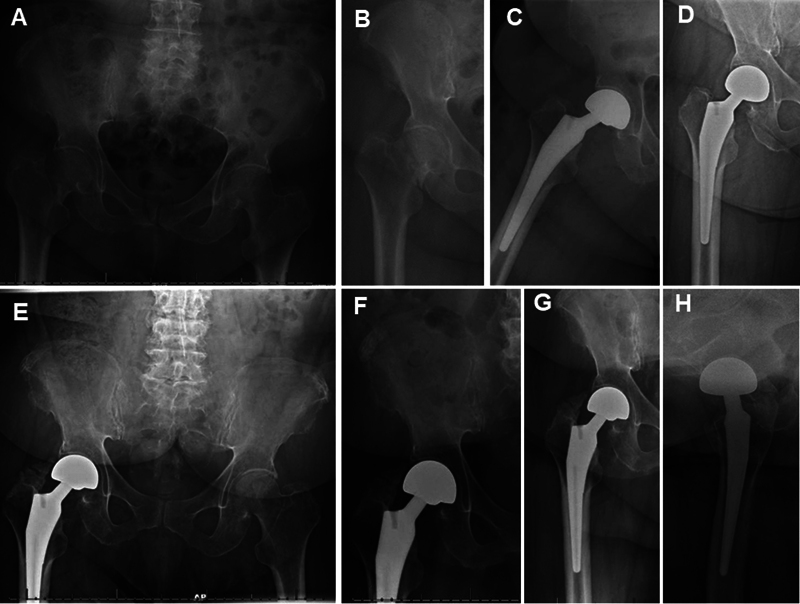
(A, B) Plain pelvic and hip radiographs of a 79.2-year-old female with multiple myeloma showing right sub capital femoral neck fracture. (C) Immediate (same day) postoperative left hip plain X-ray showing cementless bipolar hemiarthroplasty. (D) 6 weeks and (E, F) 56-month, and (G, H) 70-month follow-up plain left hip radiographs showing stable, well-fixed components without evidence of acetabular erosion. Note the presence of grade-III heterotopic ossification.
